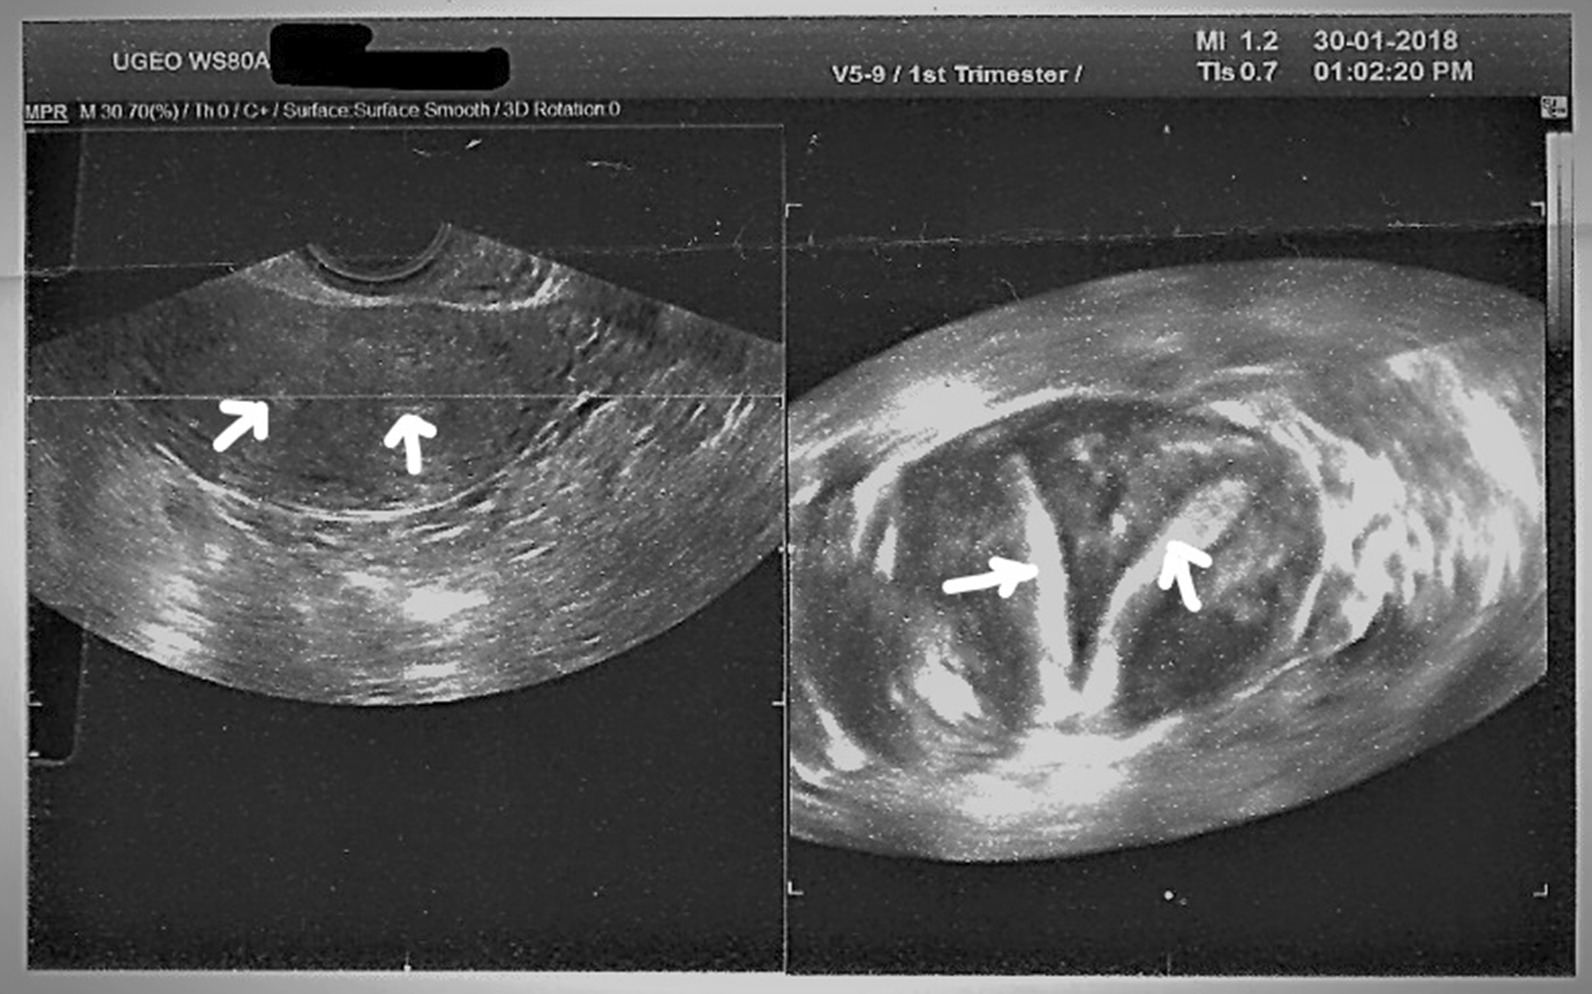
3D ultrasound of uterus didelphys in our clinical case at the time of presentation. The arrows are pointing to the two endometrial cavities of each of the two horns
